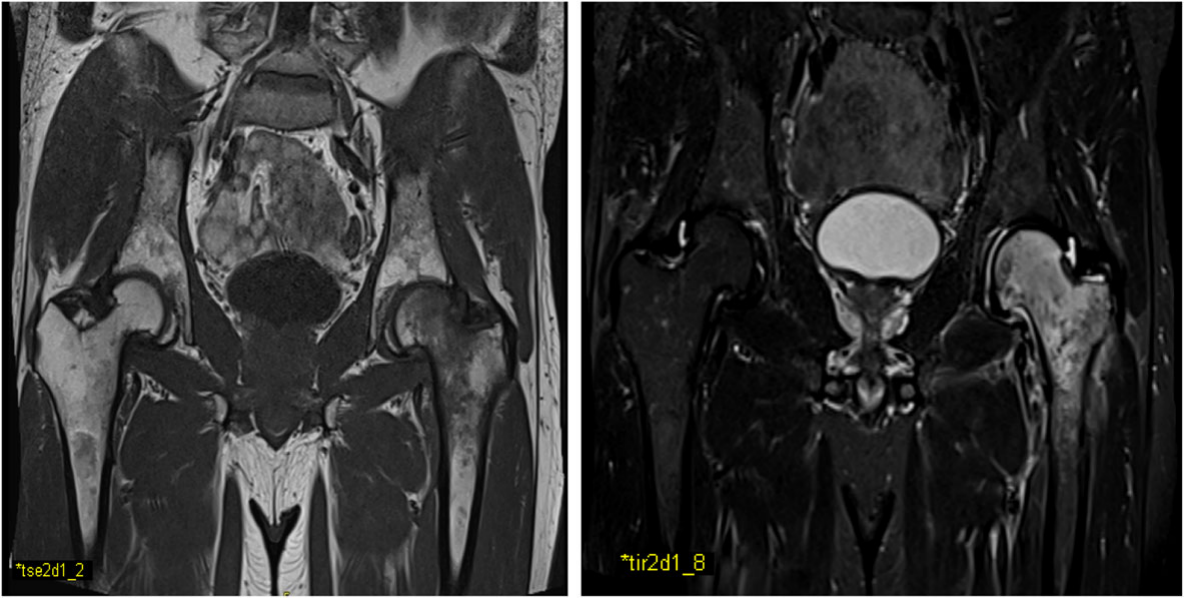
Left lower limb MRI showing remarkable and diffuse bone marrow edema
